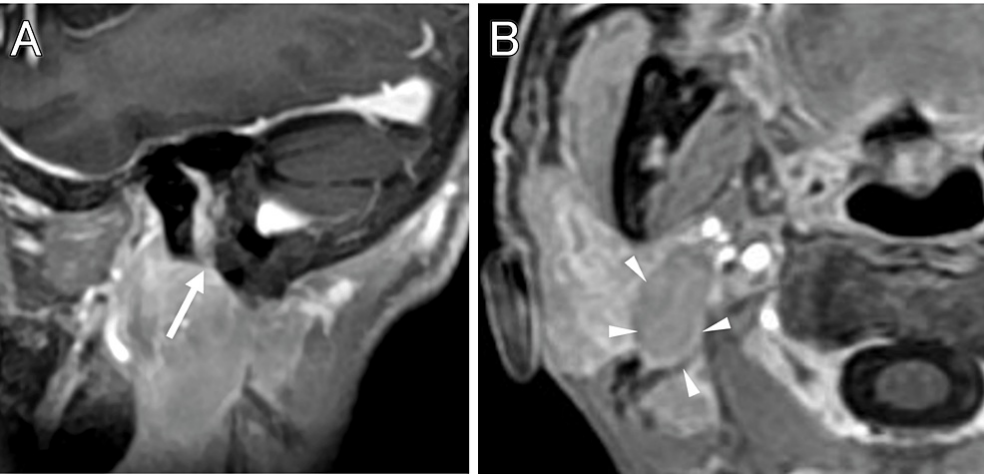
Follow-up MRI showing tumor extension to the parotid gland Contrast-enhanced T1-weighted image shows that the tumor has passed through the mastoid segment (C) (arrow) and reached the parotid gland (D) (arrowheads). MRI: magnetic resonance imaging
